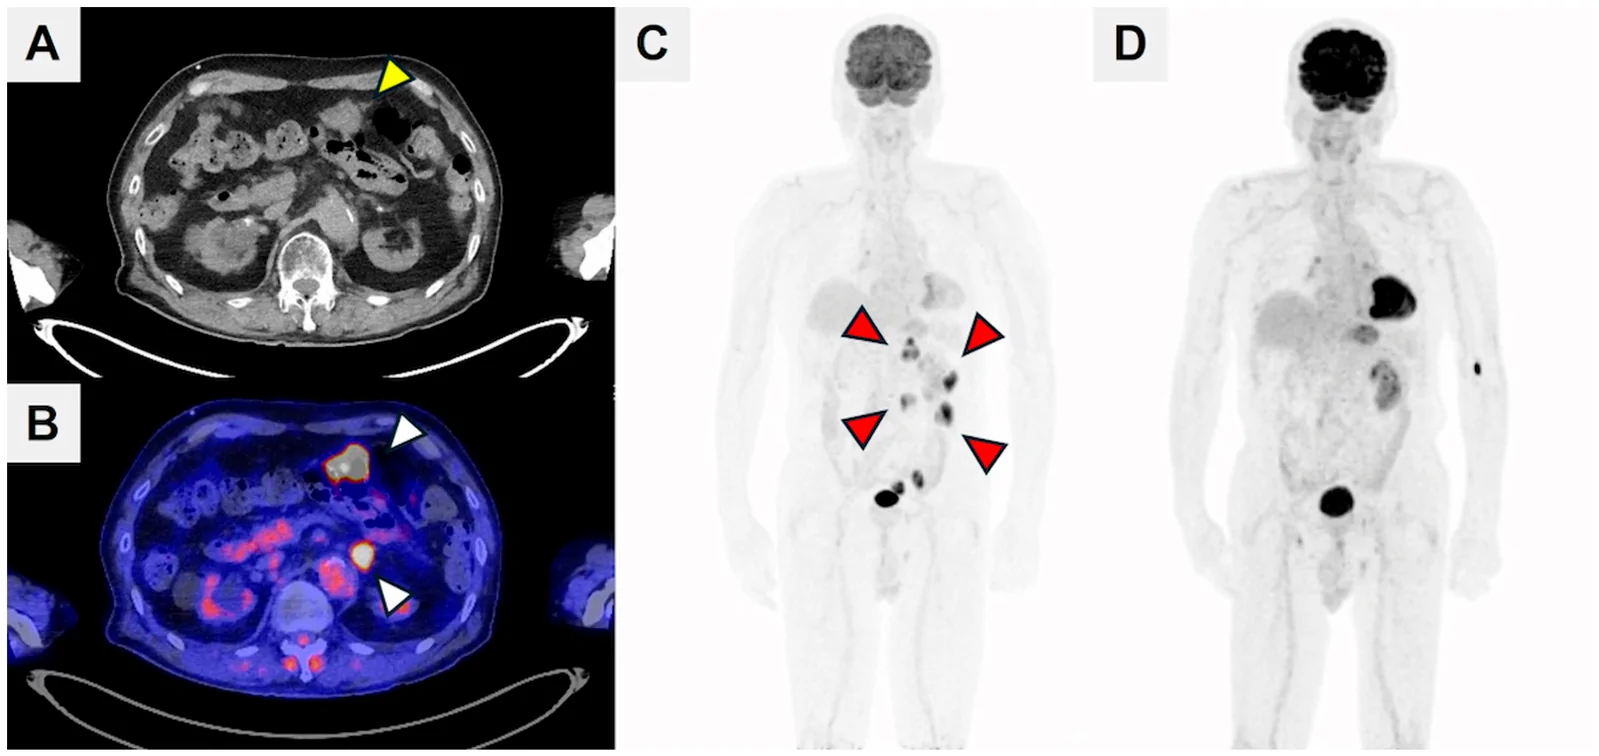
Multiple intra-abdominal masses are identified on CT imaging (yellow arrow, (A)) and FDG-PET/CT (SUVmax: 21.76 in the superior lesion and 22.31 in the posterior lesion; white arrows, (B)). MIP imaging highlights multiple FDG-avid lesions at the time of second relapse (red arrows, (C)), which completely resolved after six cycles of Pola-R therapy (D).
